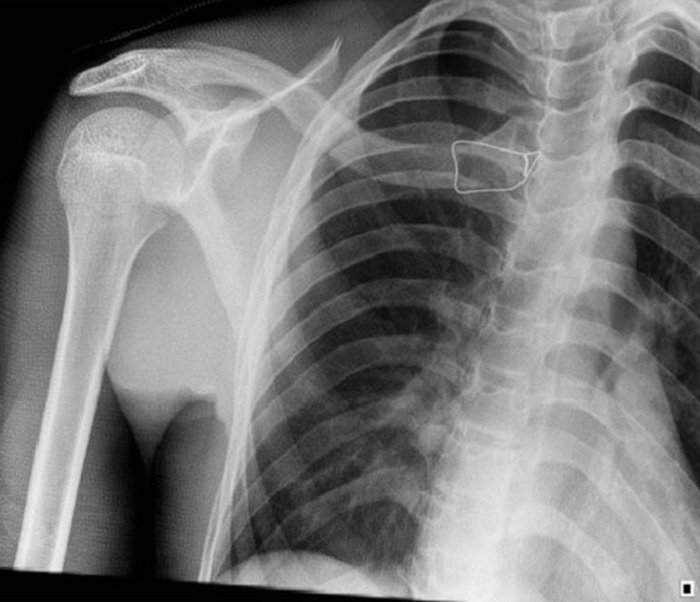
four weeks postoperative X-ray
